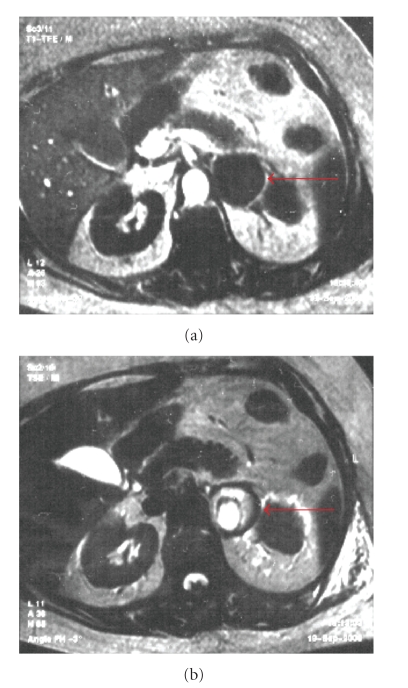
Abdominal MRI showing a large left suprarenal mass (red arrows). Right suprarenal is not seen in this view, but it was normal.
